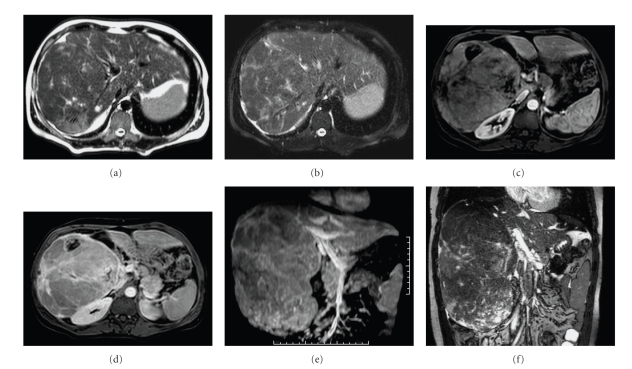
Magnetic Resonance Imaging (MRI): axial T2-weighted images (a); axial T2-SPAIR weighted image (b); axial and coronal THRIVE (c–e); coronal balanced (f); T2-weighted images demonstrated a 23 × 14 × 13 cm heterogeneous mass in the right lobe (a-b). THRIVE images showed heterogeneous contrast enhanced with enhancement of fibrosis bands in tardive-phase (c–e). This heterogeneous mass occupied almost the whole right lobe of the liver presenting a well-defined capsule (f).
